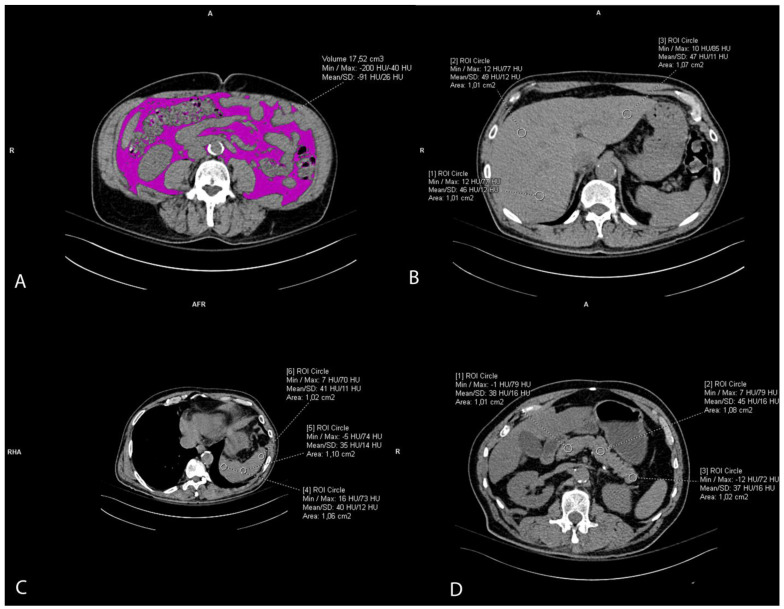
Representative axial CT images showing visceral fat volume estimation (A), liver attenuation measurement (B), spleen attenuation measurement (C), and pancreas attenuation measurement (D).
